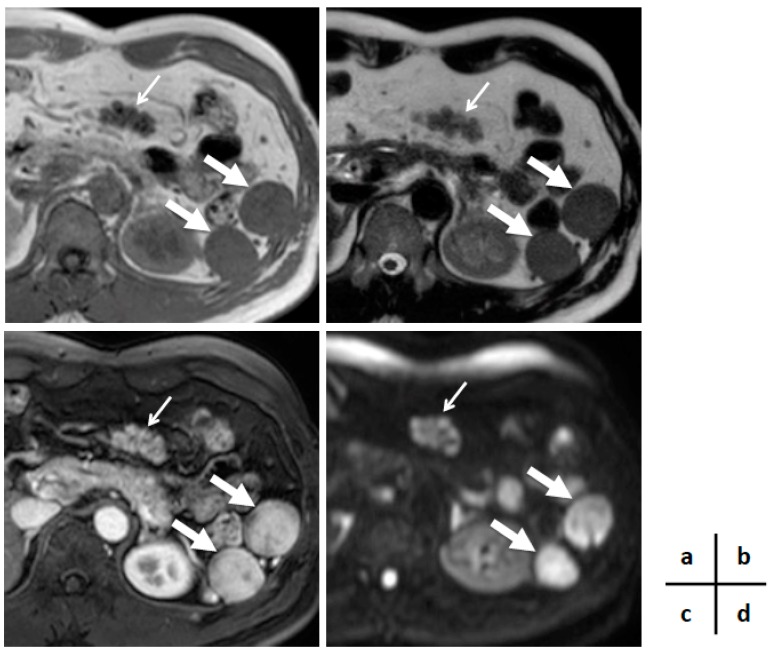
Magnetic resonance imaging (MRI). T1-weighted image (a) and T2-weighted image (b) demonstrating a low-intensity signal similar to that of the spleen. Enhanced MRI showing a similar enhancement level in the lesion and the spleen (30 s after contrast injection) (c). Diffusion-weighted image showing a mass lesion with a heterogeneous diffusion level among multiple nodules (d) (thin arrows indicating the lesion and thick arrows indicating the original spleen).
